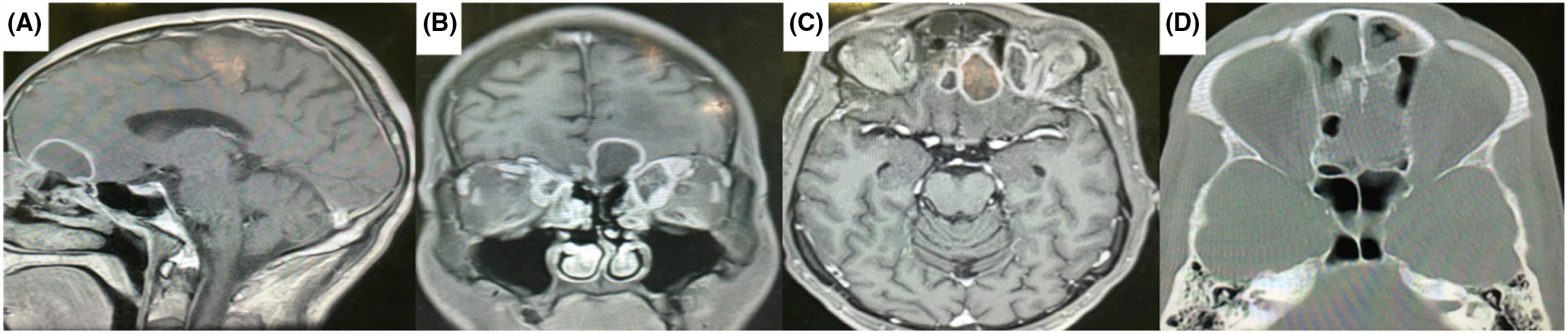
(A–C) Hypointense lesion in T1 with ring enhancement in left anterior skull base and frontal base which spreads to the medial part of left orbit (D) ethmoidal erosion in bone window CT scan.
